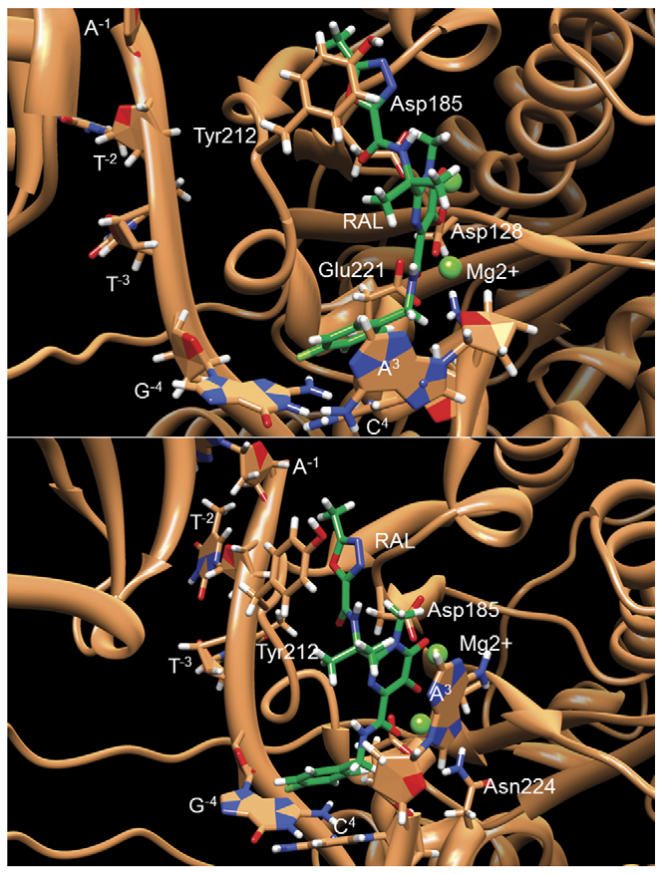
Details of the interactions of RAL with its surrounding amino acids and nucleotides as observed in the two X-ray structures of RAL bound to the PFV intasome (pdb codes: 3L2T and 3OYA). RAL is shown in slime green and IN and LTR residues in sandy brown. The amino acids and nucleotides giving interactions are shown in sticks with hydrogens in white, nitrogens in blue and oxygens in red. Mg^2+^ ions are represented by light green balls. The main difference between 3L2T and 3OYA structures concerns the orientation of the adenine A3.
